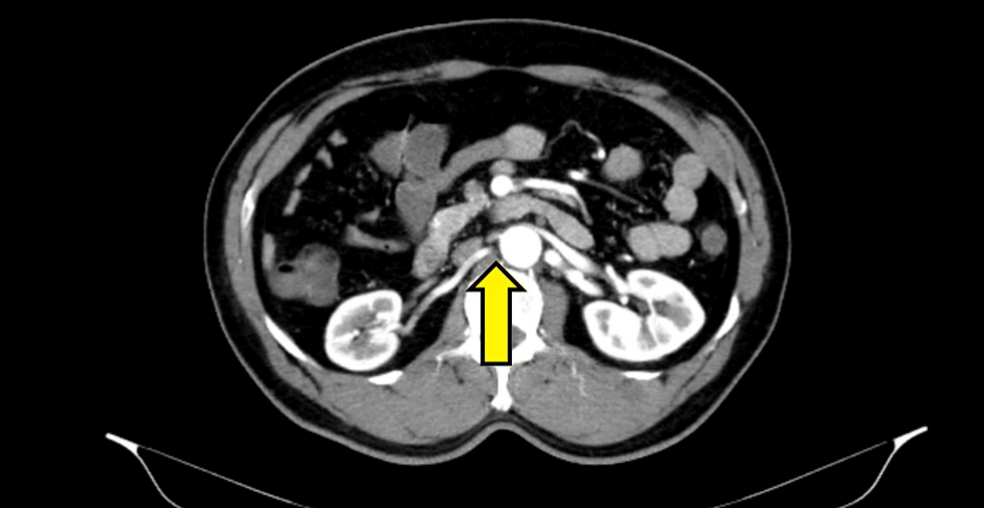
CT aortogram displaying right renal artery stenosis (yellow arrow)
